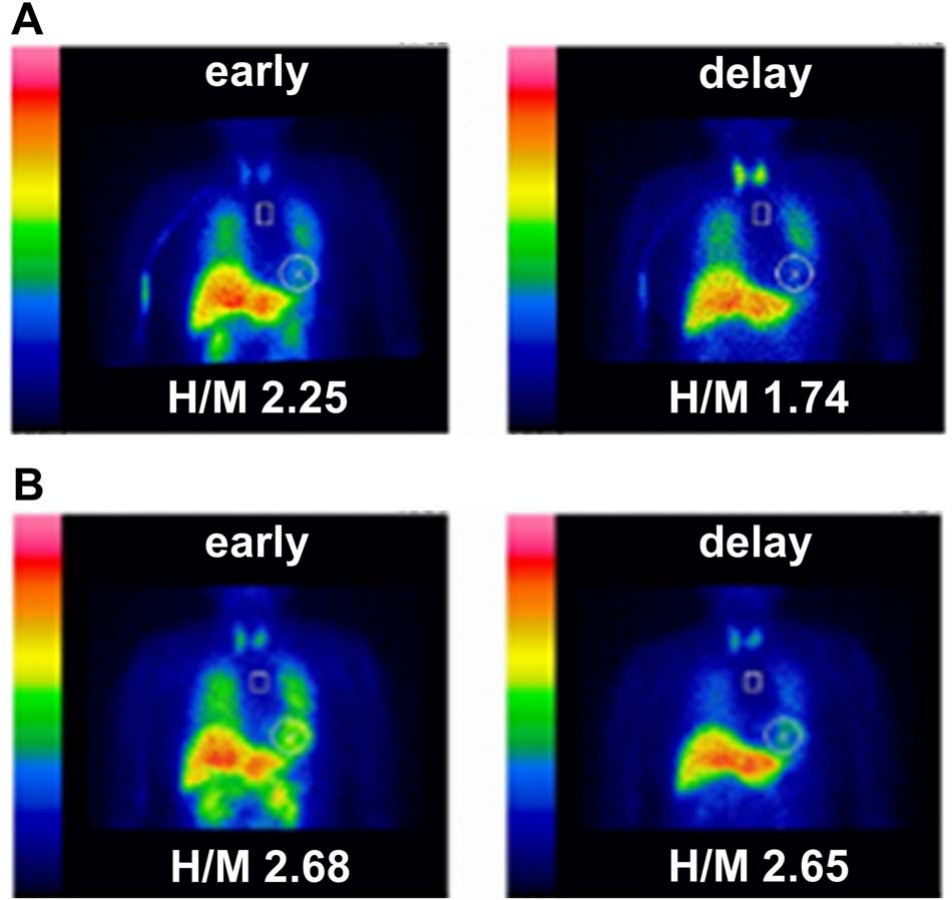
(A) ^123^I-meta-iodobenzylguanidine (MIBG) myocardial scintigraphy performed under selegiline treatment. The image shows decreased cardiac uptake with a heart-to-mediastinum (H/M) ratio of 2.25 (early value) and 1.74 (delay value). This reduced uptake is consistent with the diagnosis of Parkinson’s disease (PD). The cut-off value for the H/M ratio is 2.20. (B) MIBG myocardial scintigraphy after the discontinuation of selegiline. The image shows normalized cardiac uptake with an H/M ratio of 2.68 (early value) and 2.65 (delay value). The normalization of the H/M ratio following the cessation of selegiline suggests that the initial reduced uptake may have been influenced by the MAO-B inhibitor.
